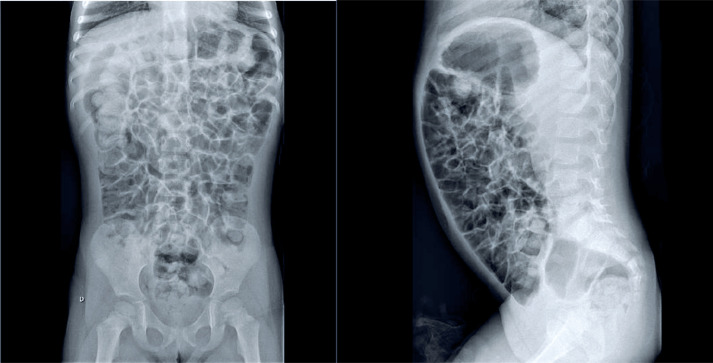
Abdominal X-rays showing mild diffuse gaseous distension of colonic and small bowel loops without pathological calcifications
